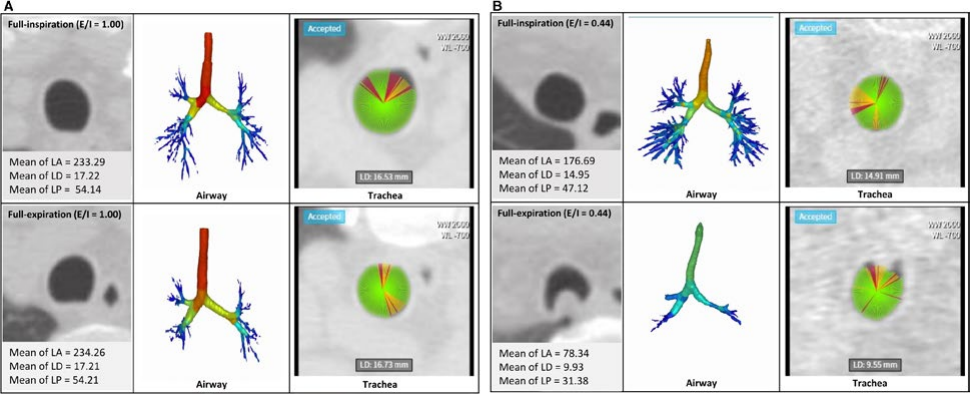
Quantitative CT measurement of trachea. Once airways were extracted and target points in the trachea were selected, quantitative tracheal parameters such as luminal diameter and wall thickness were automatically measured. Full-expiration and full-inspiration images in a healthy participant (A) showed the normal range of the change in shape and in luminal area, diameter and perimeter; expiratory-to-inspiratory (*E*/*I*) ratio of tracheal lumen area = 1.00. Full-expiration and full-inspiration images in a participant with expiratory central airway collapse (B) showed the change in shape and decrease in tracheal luminal area, diameter, and perimeter;* E*/*I* ratio of tracheal lumen area = 0.44. LA, tracheal luminal area; LD, tracheal luminal diameter; LP, tracheal luminal perimeter
